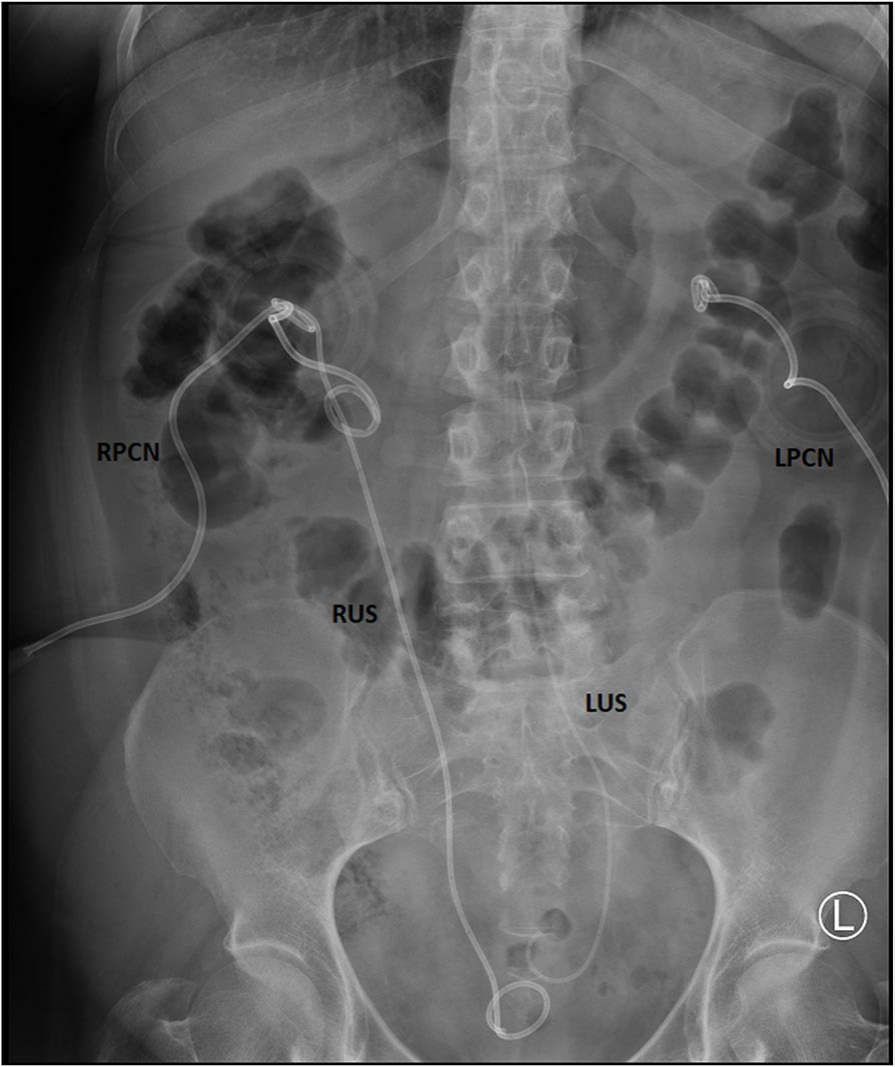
Plain radiography of the urinary tract shows a malposition of the left ureteral stent. LPCN left percutaneous nephrostomy; LUS left ureteral stent; RPCN right percutaneous nephrostomy; RUS right ureteral stent
